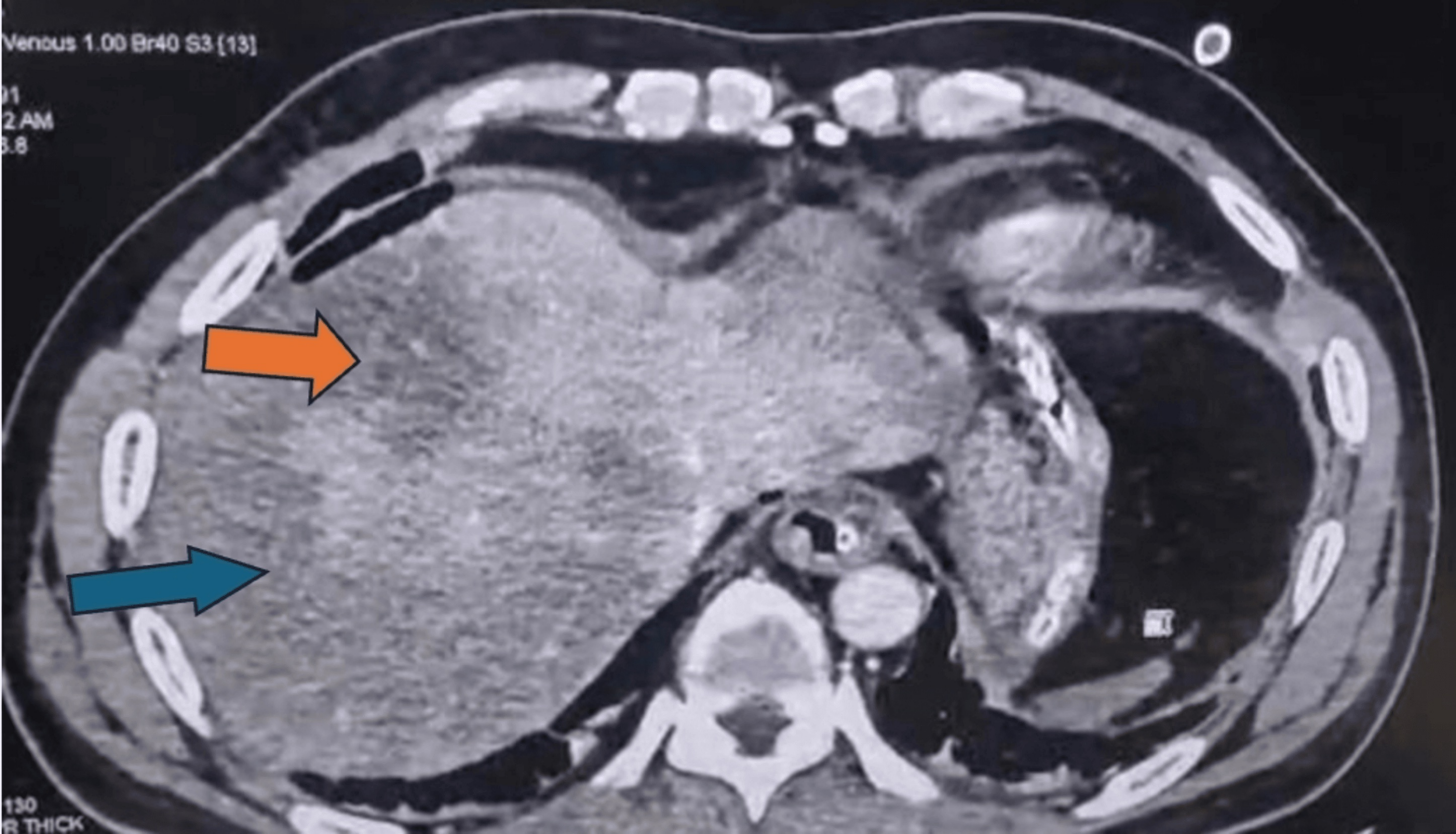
Axial contrast-enhanced computed tomography (CECT) image showing hepatic and splenic infarcts The orange arrow highlights diffuse hypoattenuation in the right lobe of the liver, while the blue arrow shows a non-enhancing, contracted spleen - both suggestive of infarcts secondary to systemic arterial thromboembolism.
